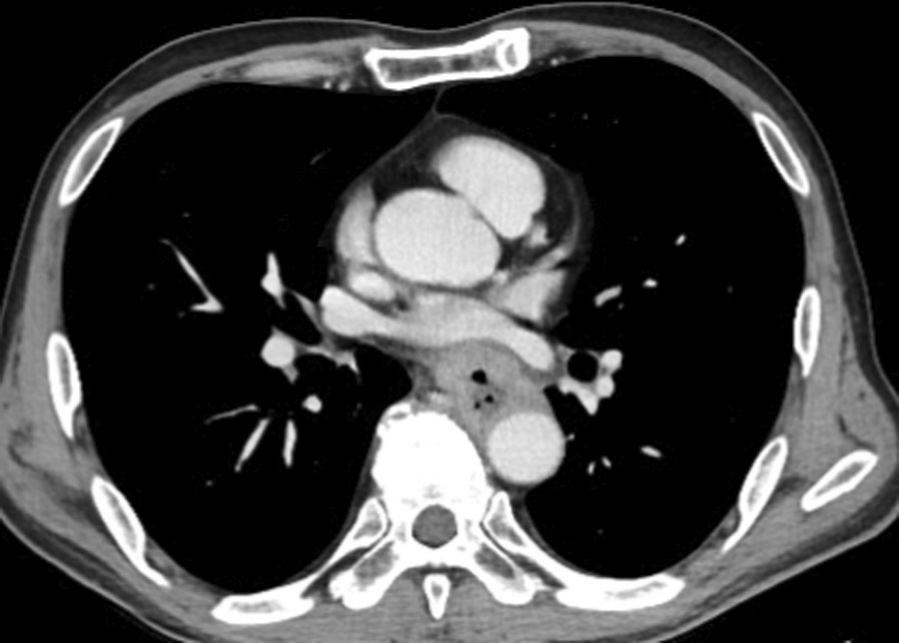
Computed tomography at 6 weeks after neoadjuvant chemotherapy showed the esophageal tumor and lymph node re-enlarged and these were extensively in continuity with the descending aorta
